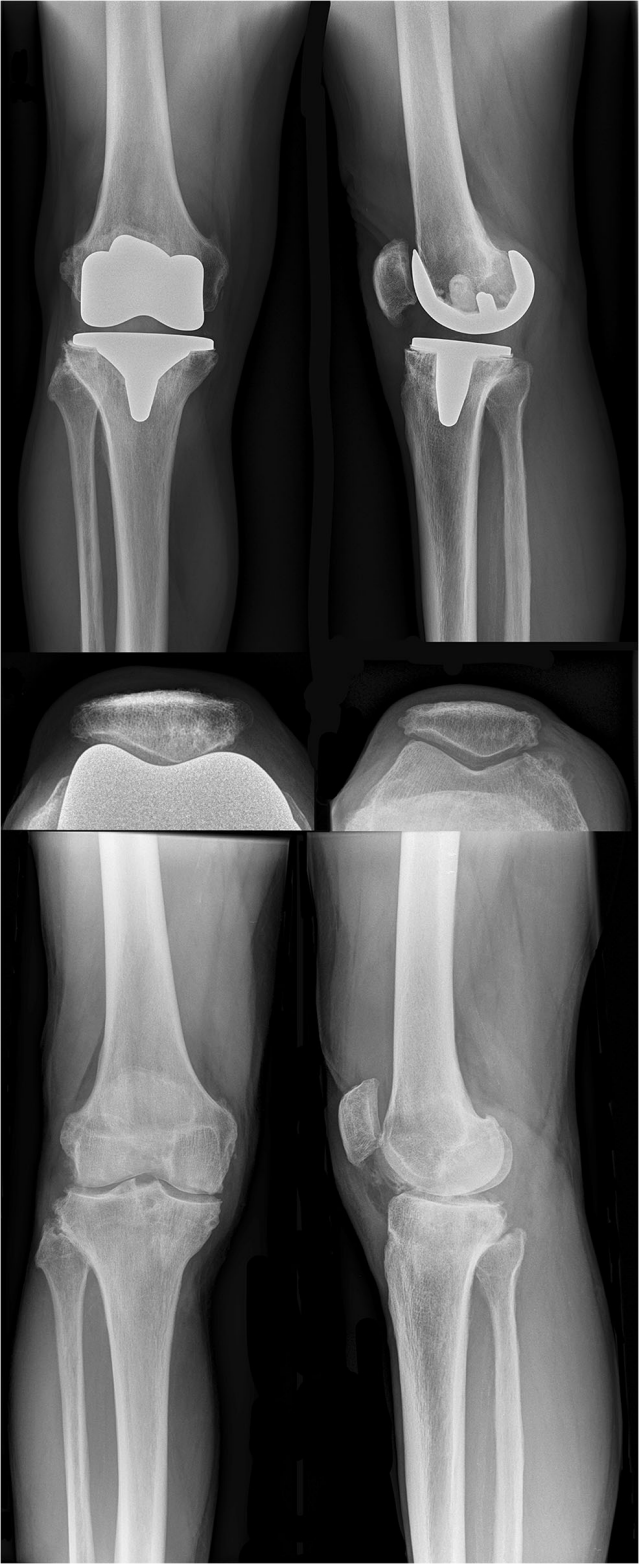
Pre- and post-operative radiographs of a patient who underwent Attune TKA. The standardised pre- and post-operative radiographs (ap, lateral, and patella tangential) of a patient who underwent Attune TKA are reported. Some differences in the femoral component with respect to the PFC can be appreciated, a shallower trochlear groove, a flatter trochlea, and the multi-radius shape of the femoral condyles. A good TKA component position and patella height are shown
